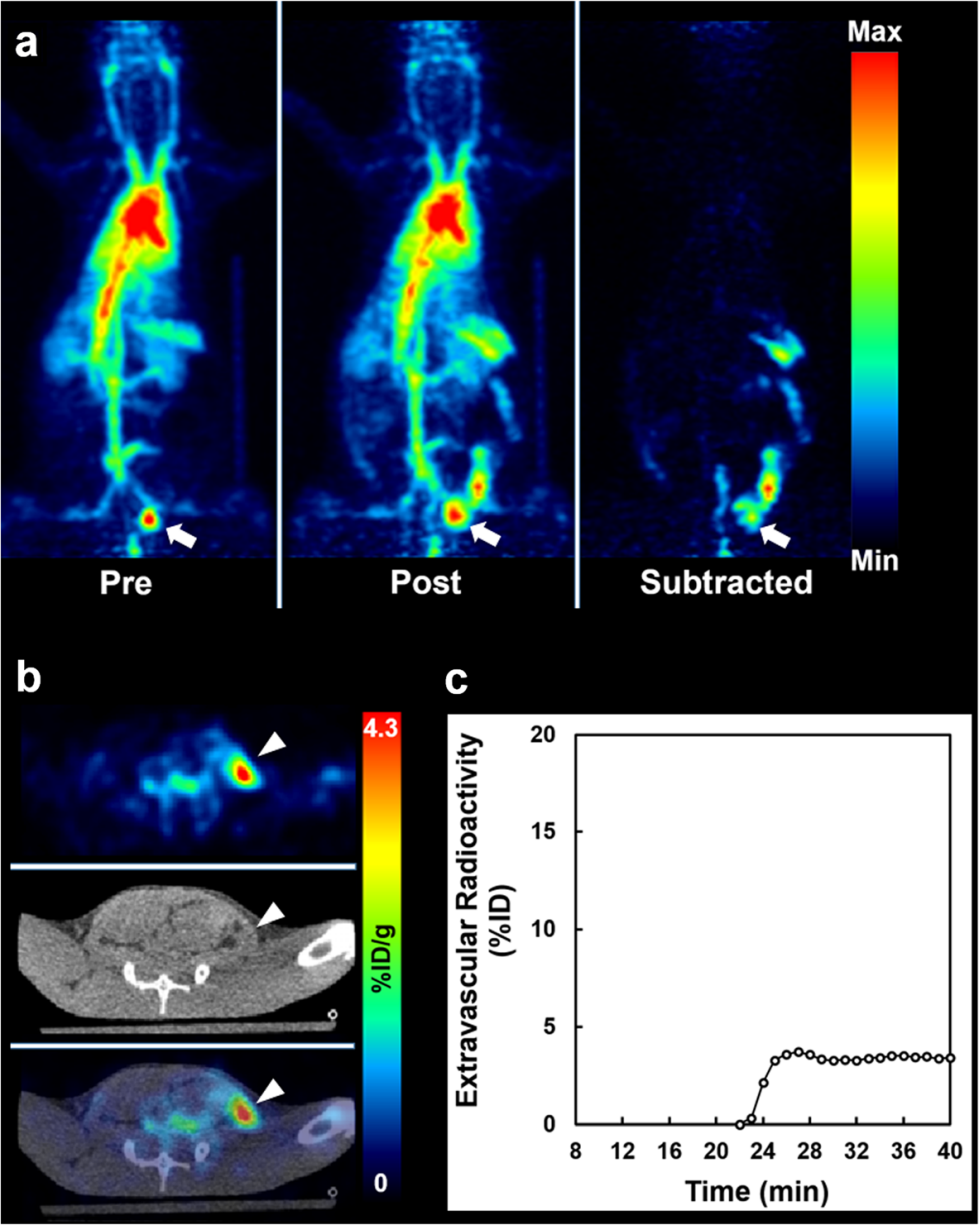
^18^F-FDG-labeled RBC PET in a temporary intraabdominal bleeding model. a Maximum-intensity-projection images in pre- (*left*), post- (*middle*) bleeding phase, and the subtracted (right) image between them. The radioactivity in the urinary bladder is seen in the images (*arrows*). b PET, CT, and fused PET/CT axial images at lower level of the abdomen. Intraabdominal bleeding is identified as high radioactivity in the extravascular space in the fused image (*arrowheads*). c Time-activity curve of extravascular radioactivity in the subtracted image. The radioactivity appeared soon after the manual puncture of the colonic wall (22 min), and then it reached a plateau about 5 min later
